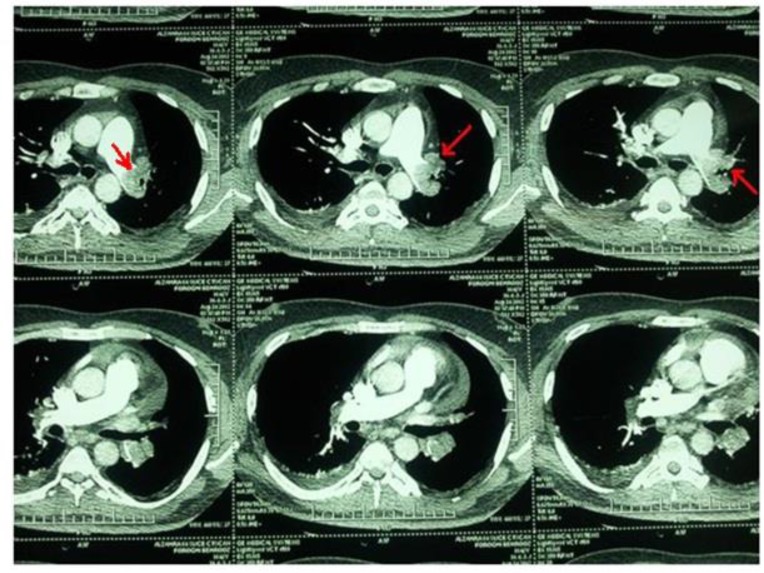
CT angiography confirmed the presence of large pulmonary thromboembolism
